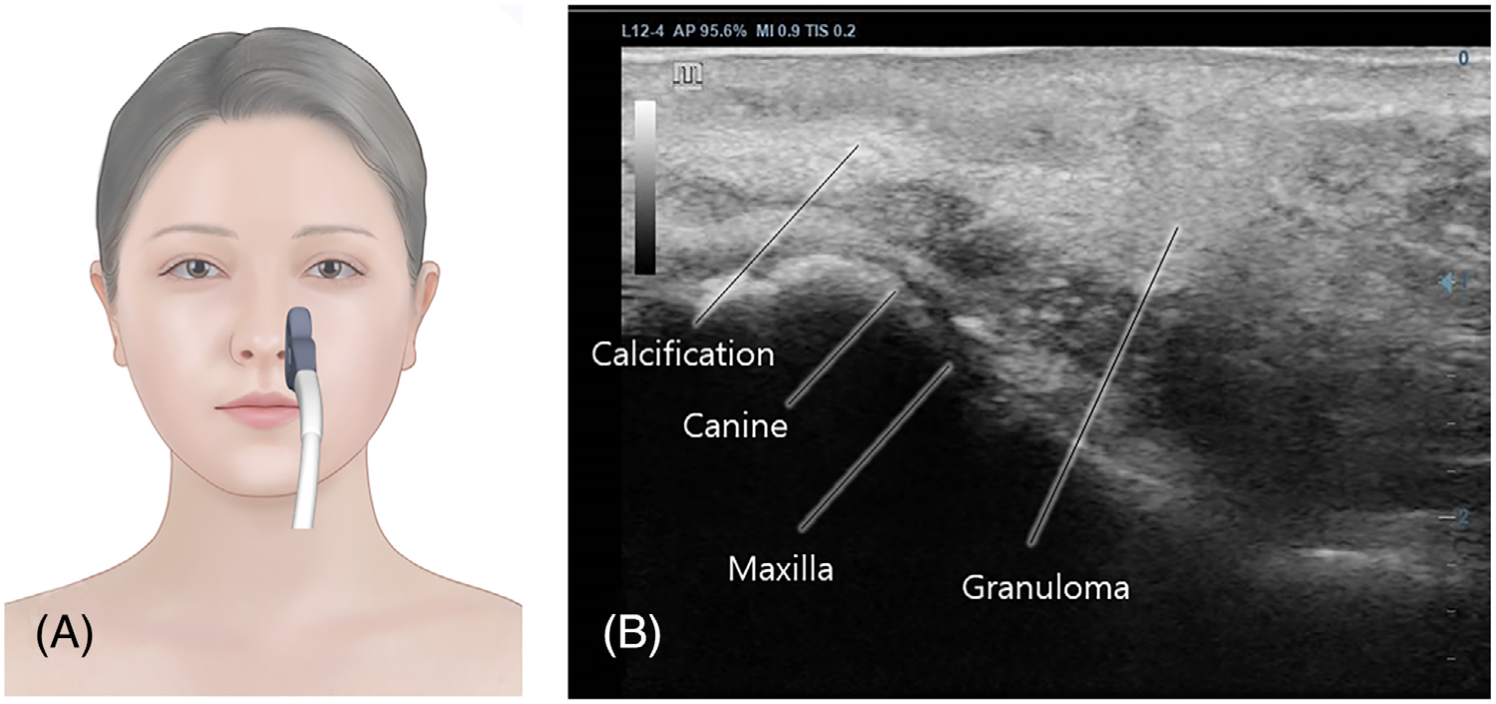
Ultrasonographic assessment of the left nasolabial fold in the longitudinal plane (A) depicted granulomas appearing as hyperechoic lesions beneath the dermal layer (B).
